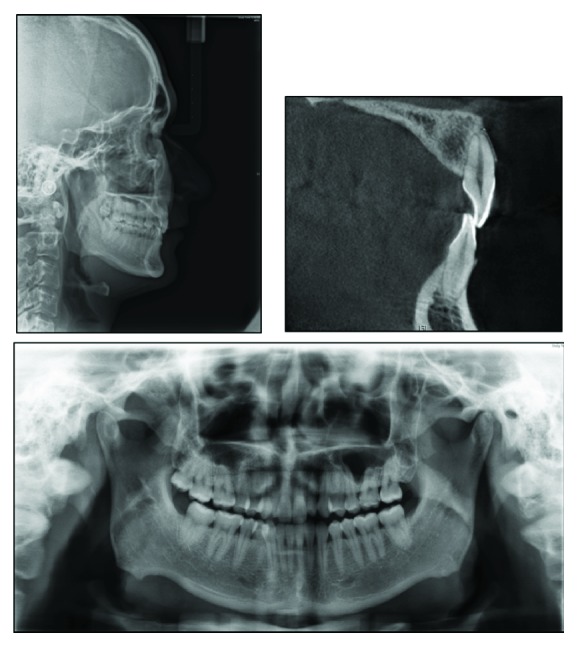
Pretreatment cephalography, panoramic radiograph, and pretreatment image of maxillary left central incisor from CBCT.
